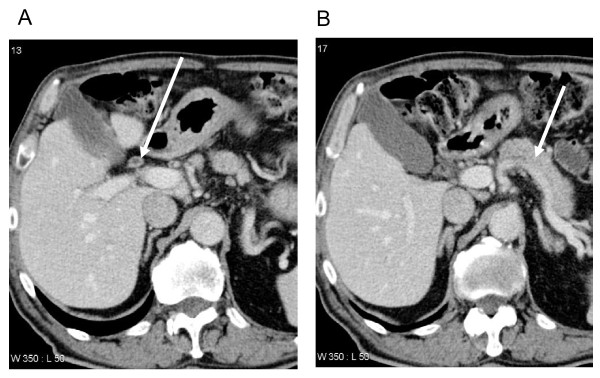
Abdominal CT scan. (A) Arrow pointing to bile duct wall thickening. (B) Arrow outlining atrophic pancreas.
